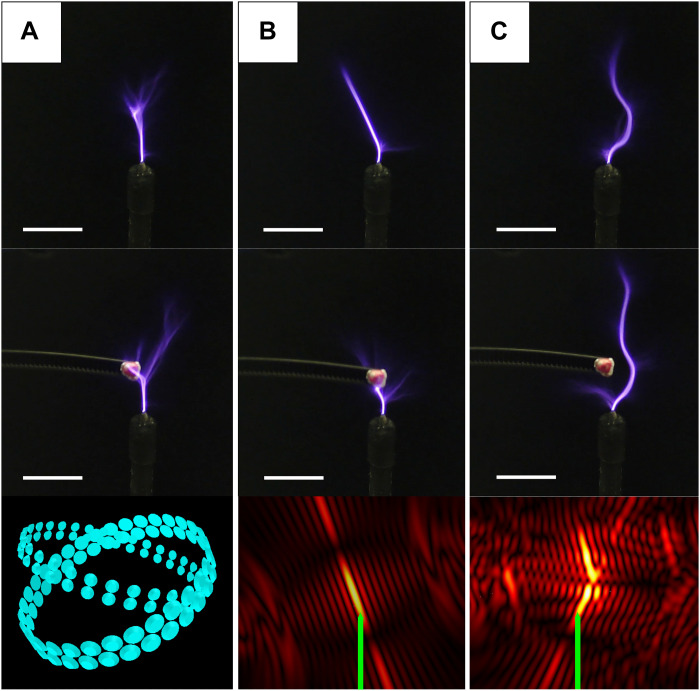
Guidance of the spark along a curved acoustic field created with two focal points coming from two rings of emitters. (A) No ultrasound. (B) Only one focal point. (C) Curved field created with two intersecting focal points. The top row shows the spark without the presence of the obstacle, middle row with a popcorn kernel, and bottom the amplitude field. The electrode is marked in green. Scale bars, 1 cm.
